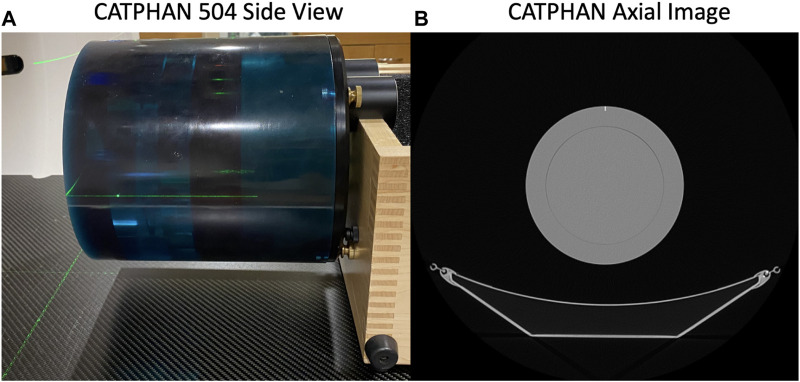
The CATPHAN 504 imaged in this study is shown (A) setup on the CT table and (B) with an axial CT image of the uniformity region.
